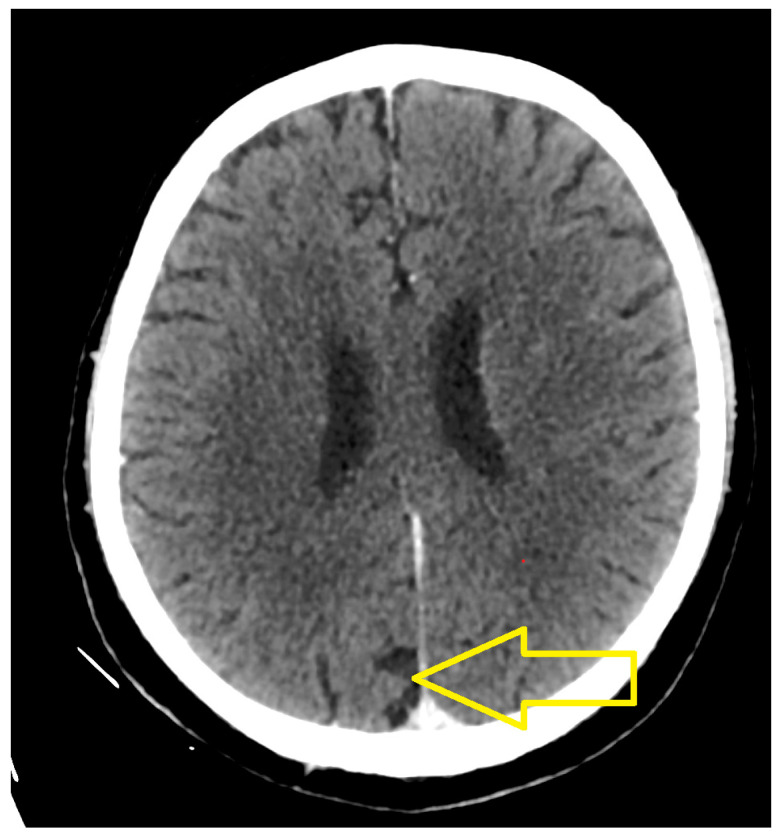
Skull CT (June 2024): At the cerebral level, in the right para-sagittal parietal vertex, a secondary brain metastasis is evident, in regression compared to March 2023.
